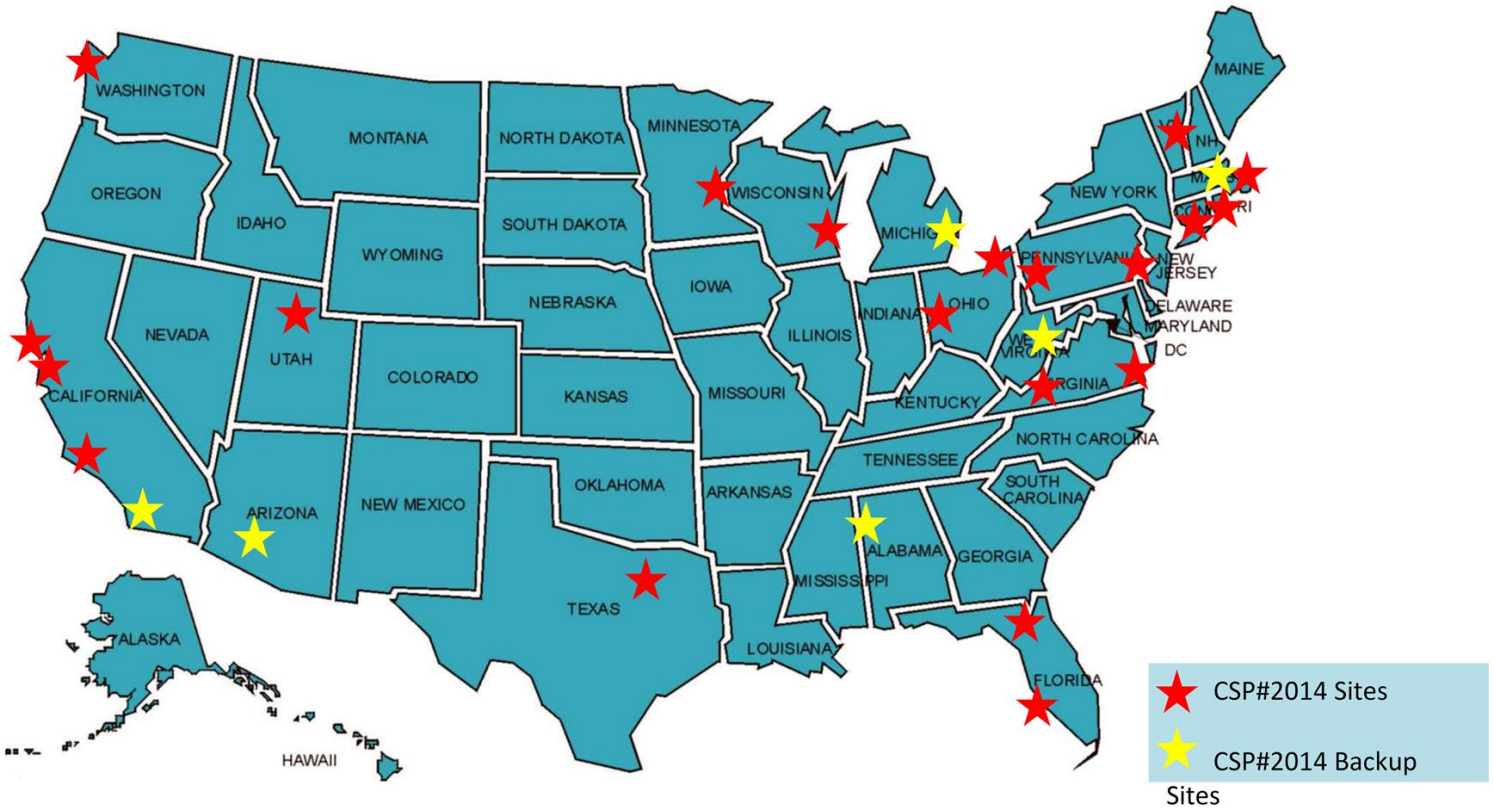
CSP2014: VA-BRAVE map of study sites. Original active sites: Bay Pines, FL; Boston, MA; Cleveland, OH; Dallas, TX; Dayton, OH; Gainesville, FL; Hampton, VA; Long Beach, CA; Milwaukee, WI; Palo Alto, CA; Philadelphia, PA; Pittsburgh, PA; Providence, RI; Salem, VA; Salt Lake City, UT; San Francisco, CA; West Haven, CT; Seattle, WA; White River Junction, VT. Back-up sites: Bedford, MA; Detroit, MI; Huntington, WV; Phoenix, AZ; San Diego, CA; Tuscaloosa, AL
